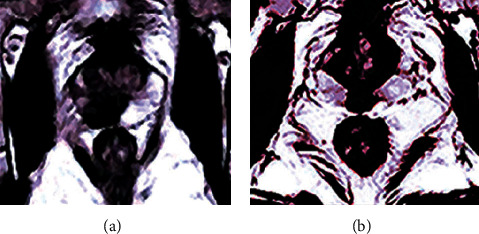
Comparison on MRI images of prostate cancer and prostate hyperplasia after optimized treatment. (a) Prostate cancer. (b) Hyperplasia of prostate.
